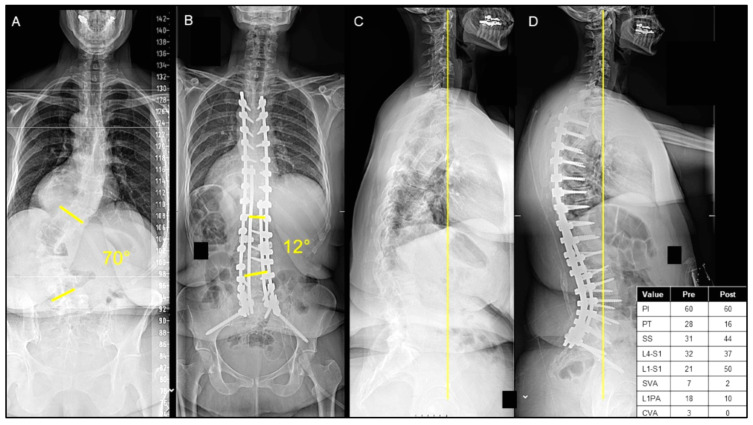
(A–D) A case presentation of a 49-year-old female presenting with adult idiopathic scoliosis and significant back pain with a significant sagittal/coronal malalignment, as seen on posterior–anterior (PA) (A) and lateral X-rays (C). The patient underwent T4 pelvis posterior spinal instrumentation with T11-L5 posterior column osteotomies, as seen on the postoperative PA (B) and lateral X-rays (D). The patient was discharged home on postoperative day 13.
